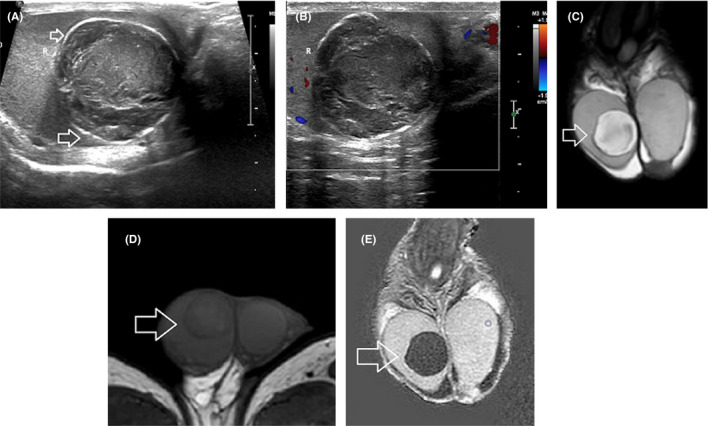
Epidermoid cyst. (A) Gray‐scale image depicts a solid, heterogeneous, intratesticular mass, of laminated appearance. The lesion is surrounded by a thin echogenic rim (small arrows). (B) Color Doppler image shows absence of internal vascularity. (C) Coronal T2WI demonstrates right intratesticular mass lesion (arrow), with heterogeneous signal, mainly hyperintense, encircled by a hypointense halo. (D) Axial T1WI demonstrates lesion (arrow) internal heterogeneity. (E) Coronal subtracted DCE image depicts absence of lesion vascularity (arrow), a finding confirming the diagnosis of benignity
